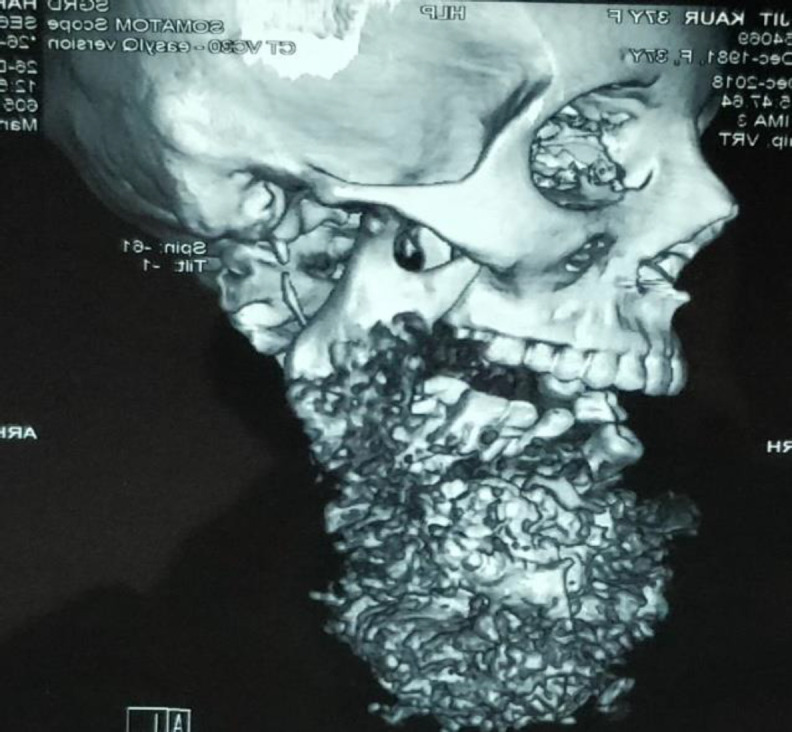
3D CT (Right) reveals soft tissue infra-condylar lesion 15*12*10cm
